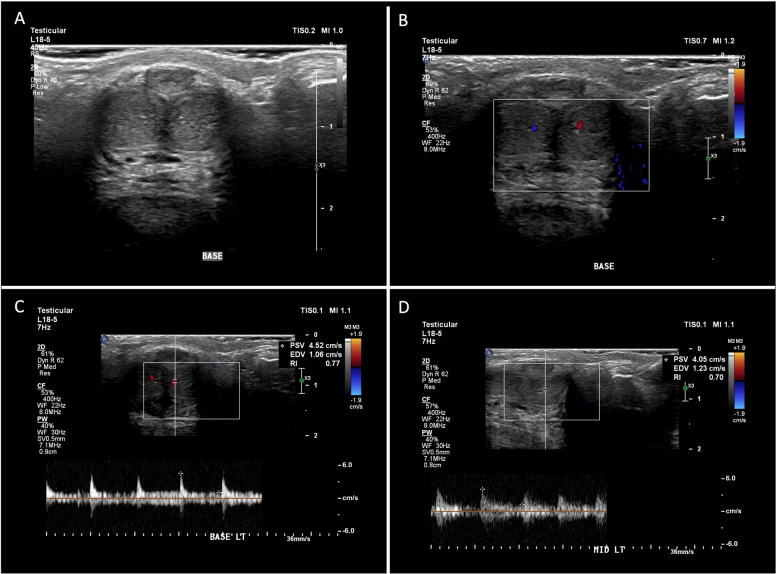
Follow-up ultrasound. A B-mode image demonstrating resolution of cavernosal haematoma. B No residual left cavernosal fistula. C, D Restoration of the normal low-flow, low resistance waveform in the proximal and mid left cavernosal arteries with a peak systolic velocity of 6 cm/sec
